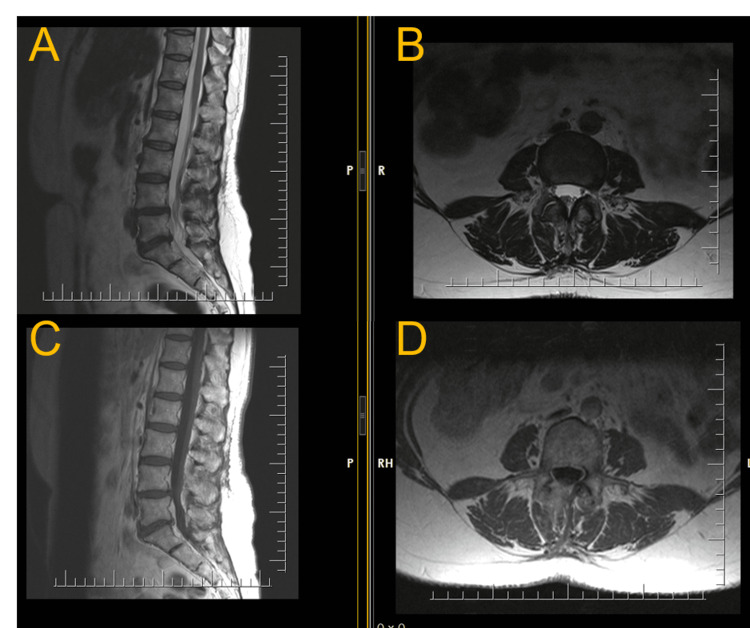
Postoperative MRI three months after surgery. Sagittal (A) and axial (B) T2-weighted images and sagittal (C) and axial (D) T1-weighted images showing no signs of the intradural mass at the level of the L4 vertebrae.
